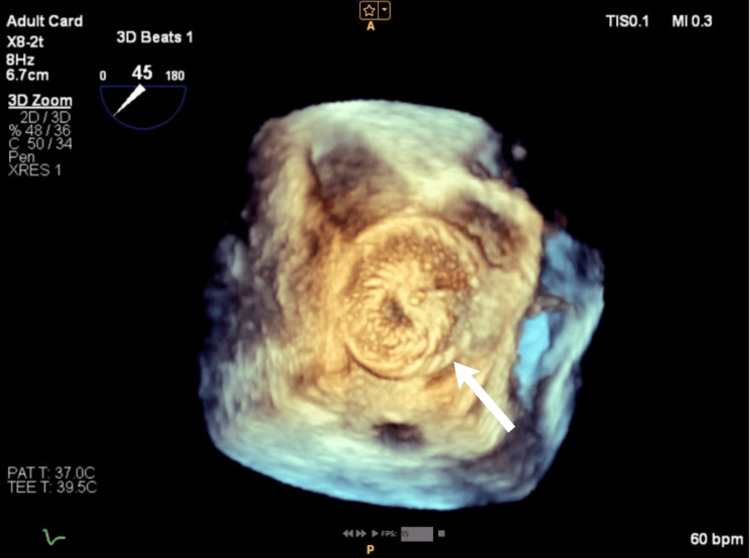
Real-time 3D TEE image of ASD II demonstrating the normal position of the device. TEE: transesophageal echocardiography; ASD II: secundum atrial septal defect
